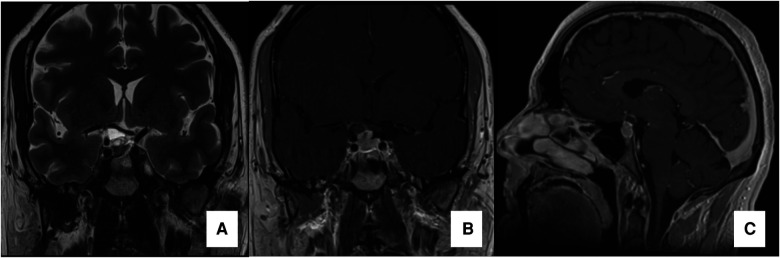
Preoperative MRI preoperative MRI. Coronal T2WI image in (A), Coronal and Sagittal T1WI images with gadolinium (B and C, respectively) demonstrating a solid-cistic sellar lesion with suprasellar extension compressing the optic chiasm (especially its right portion) and promoting a superior displacement of the first segment of the right anterior cerebral artery.
